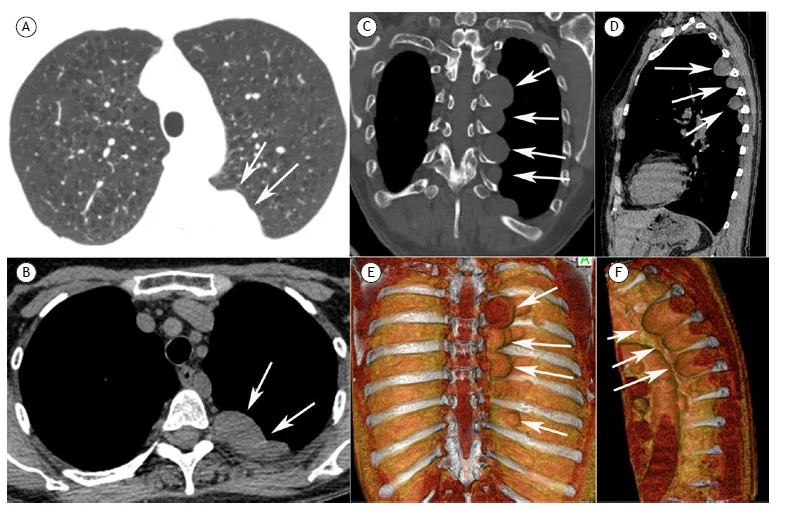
(A) Axial chest CT image showing numerous small, rounded, emphysematous cysts in both lungs. Axial (B), coronal (C), and sagittal (D) reconstructions with mediastinal window settings demonstrating paravertebral and intercostal soft-tissue masses involving the left neural foramina at multiple vertebral levels, with extension along intercostal spaces, following the courses of corresponding thoracic nerve roots and intercostal nerves (arrows). Coronal (E) and sagittal (F) three-dimensional volume-rendered CT reconstructions show the spatial relationships among the masses, vertebral bodies, and posterior ribs.
